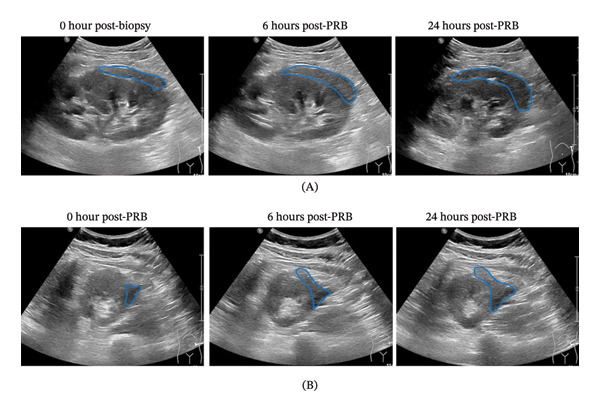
Ultrasound imaging of hematomas postpercutaneous renal biopsy.
